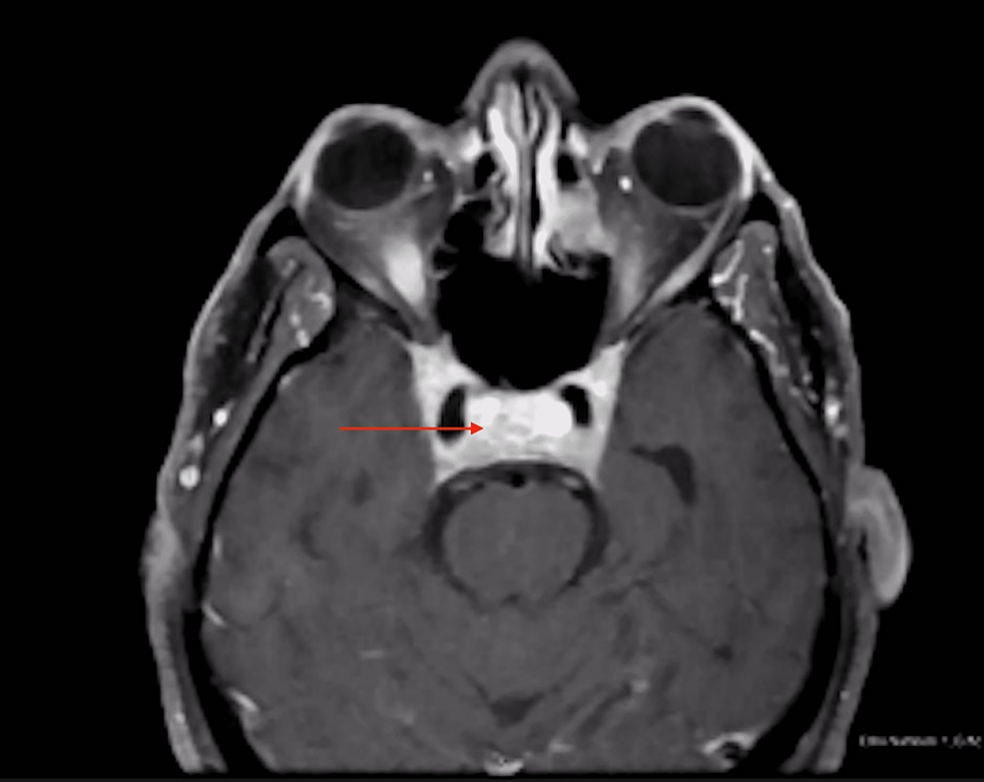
Post-contrast T1 Fat-Sat MRI Brain Demonstrating Diffuse Abnormal Thickening and Enhancement of the Orbital Apices, Superior Orbital Fissures, Cavernous sinuses, and Sella
